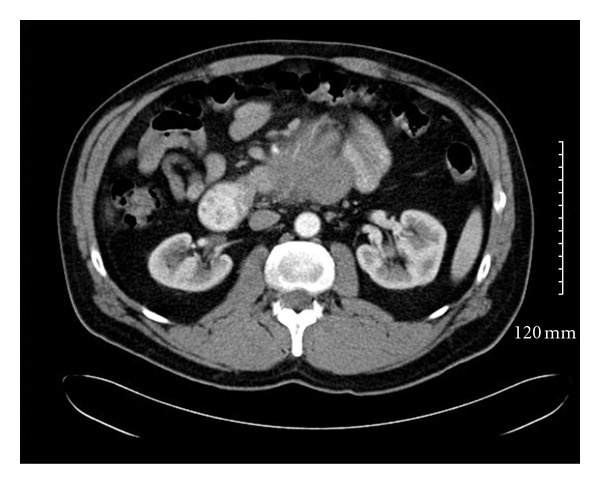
Initial CT abdomen with contrast showing a diffuse 6.1 cm × 5.9 cm × 8 cm thickening of the wall of duodenum with adjacent encasement of the superior mesenteric artery.
